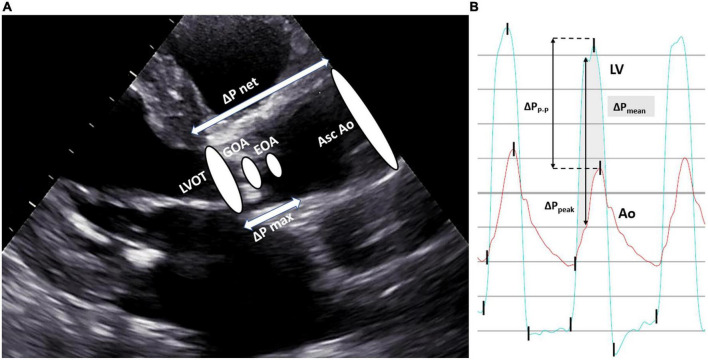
Echocardiographic and invasive characterization of aortic stenosis. Panel (A) The geometric orifice area (*GOA*) is the true anatomical area of the aortic valve and the area of the flow jet at the vena contracta, which occurs downstream of the valve orifice, is the effective orifice area (*EOA*), and corresponds to the calculated AVA by the continuity equation. GOA is always larger than EOA (they will be equal if GOA has the same size as LVOT). The pressure difference between the LVOT and EOA is known as ΔP_max_. The pressure difference between the ascending aorta and LVOT is ΔP_net_, as it is recorded after the occurrence of pressure recovery, and corresponds to the measured pressure drop in the catheterization laboratory. In the presence of the pressure recovery phenomenon, ΔP_max_ is higher than ΔP_net_, which partially explains the discrepancies between Doppler and invasive metrics. Panel (B) The shaded area represents the mean transaortic pressure drop (ΔP_mean_); peak-to-peak pressure drop (ΔP_P–P_) is the difference between the peak LV pressure and the peak aortic pressure at two different points in time; maximum instantaneous pressure drop (ΔP_peak_) is the maximum recorded difference between the LV and aortic pressure at the same point in time. *Ao indicates aortic pressure; Asc Ao, ascending aorta; LV, left ventricle pressure; LVOT, left ventricular outflow tract.*
